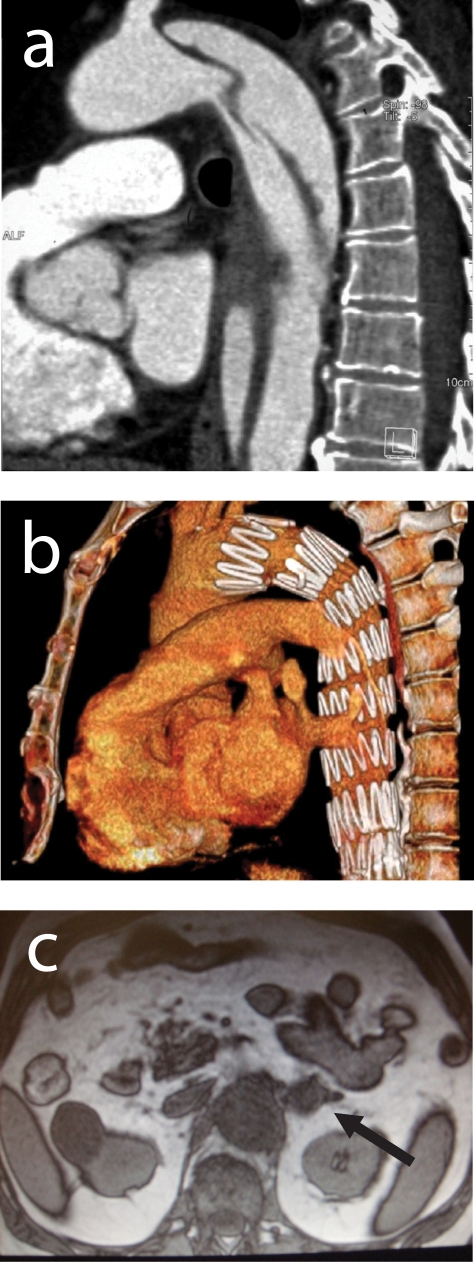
(a) A CT scan of thorax and abdomen demonstrated a dissection starting just below left succlavian artery and extending downward to the left renal artery, involving the celiac tripod and superior mesenteric artery. (b) A postoperative control CT scan demonstrated the correct graft positioning, not endoleaks and the closure of all secondary tears. (c) A MRI of abdomen showing a left adrenal lesion of 2 cm of diameter.
